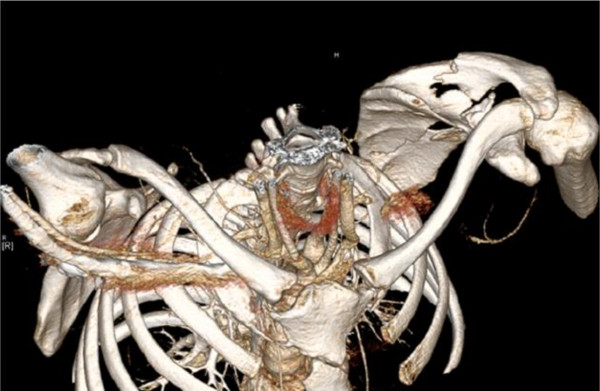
CT-3D-reconstruction of shoulder girdle. Visualization of displaced scapula fracture on the left side. Note also the extravasation of contrast agent and the missing presentment of the ipsilateral subclavian artery.
